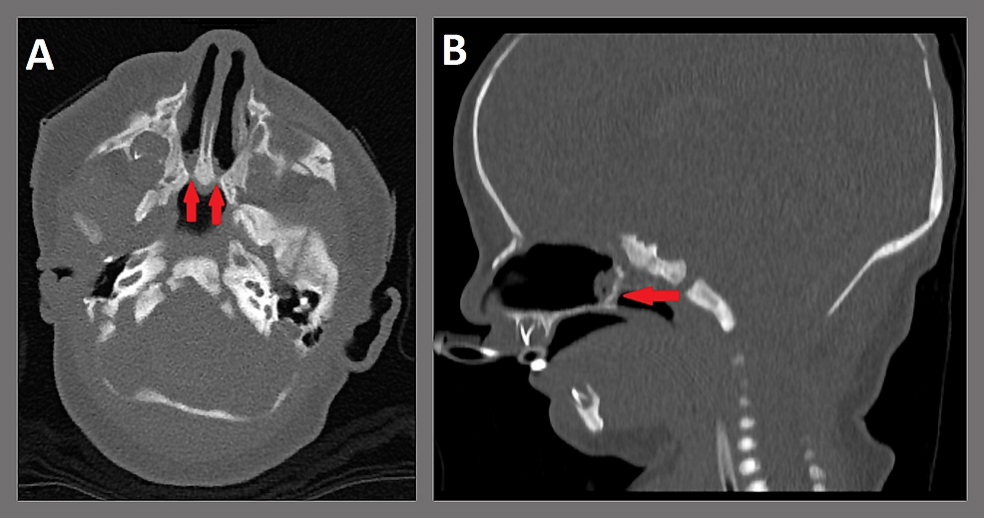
CT landmark sinus protocol without contrast (A: axial plane; B: sagittal plane). Note: Bilateral mixed membranous and bony atresia with a choanal size of less than 1 mm in diameter (red arrows).
